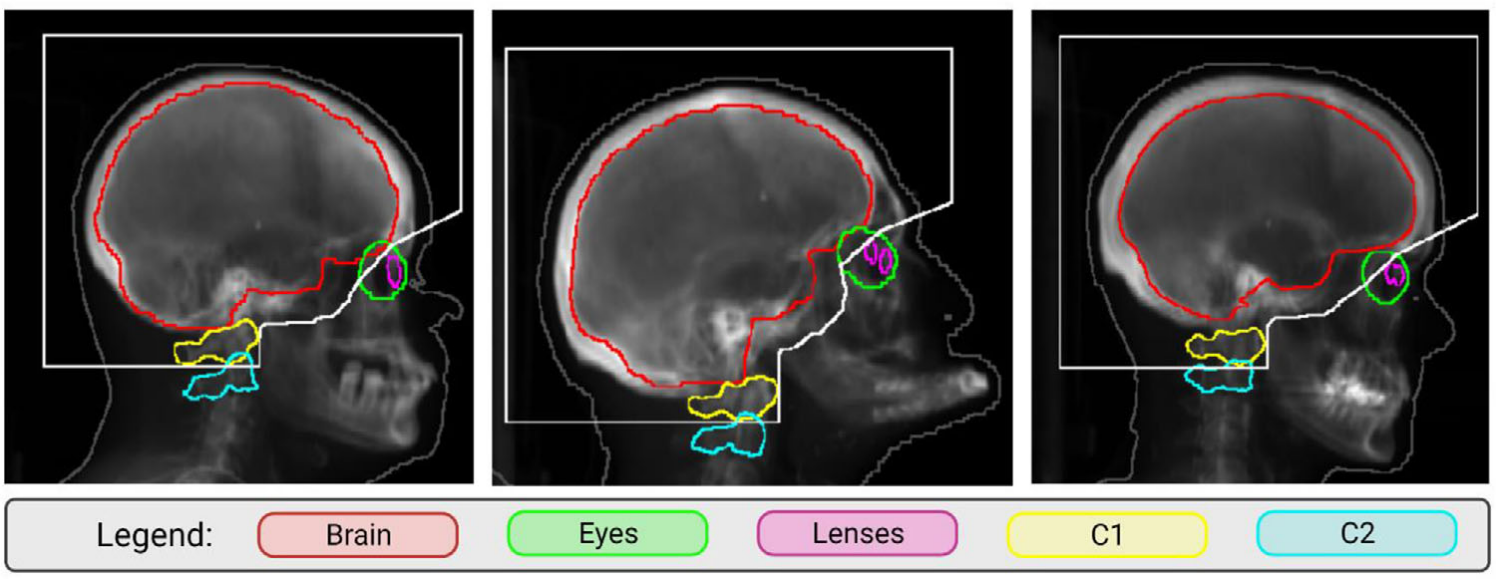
Examples of landmark‐based apertures (outlined in white) on digitally reconstructed radiographs for three patients
